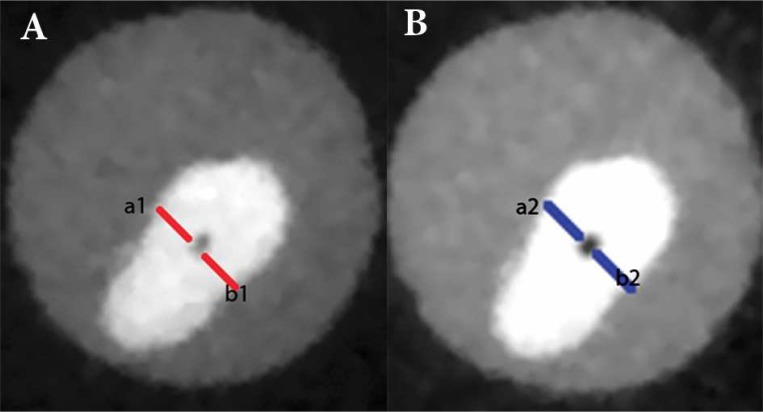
CBCT images at 5-mm distance from the apex; *A)* pre- and *B)* post-instrumentation images with Neoniti. Amount of canal transportation was obtained from (*a*_1_*-a*_2_)-(*b*_1_*-b*_2_) formula
